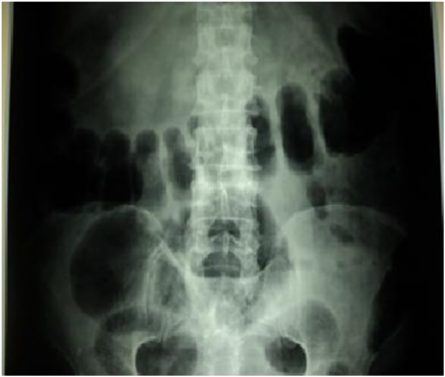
Supine abdominal plain film showing air-filled distended gut loops.
